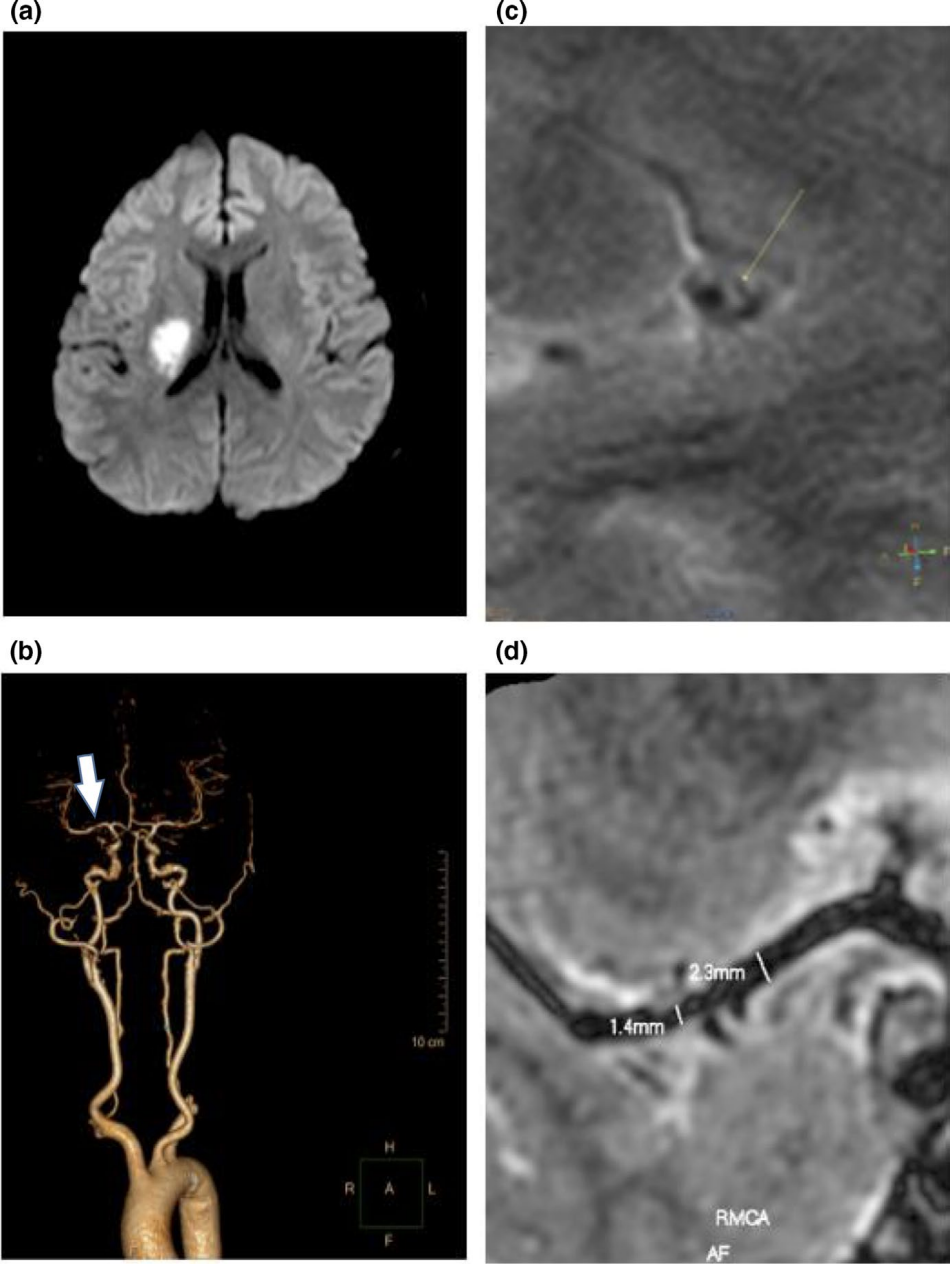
Parent artery atherosclerosis occluding penetrating artery (POPA) stroke mechanism in ischemic stroke patients with ICAS. Images from a 50‐year‐old man with acute cerebral infarction in the right basal ganglia. When the patient was admitted to the hospital, the consciousness was clear, presented with the left side of the nasolabial fold became shallow, the left upper limb muscle strength was level 2, the left lower limb muscle strength was level 5‐, and the right limb muscle strength was level 5. NIHSS on admission was 3, and mRS at discharge was 2. (a) An isolated acute infarction in penetrating artery territory indicating probable parent artery atherosclerosis occluding penetrating artery. (b) CTA showed stenosis in M1 segment of right middle cerebral artery. The arrows indicate stenosis location. (c d) High‐resolution magnetic resonance showed plaque in M1 segment of right middle cerebral artery blocks vessels and leading to 60% lumen stenosis
